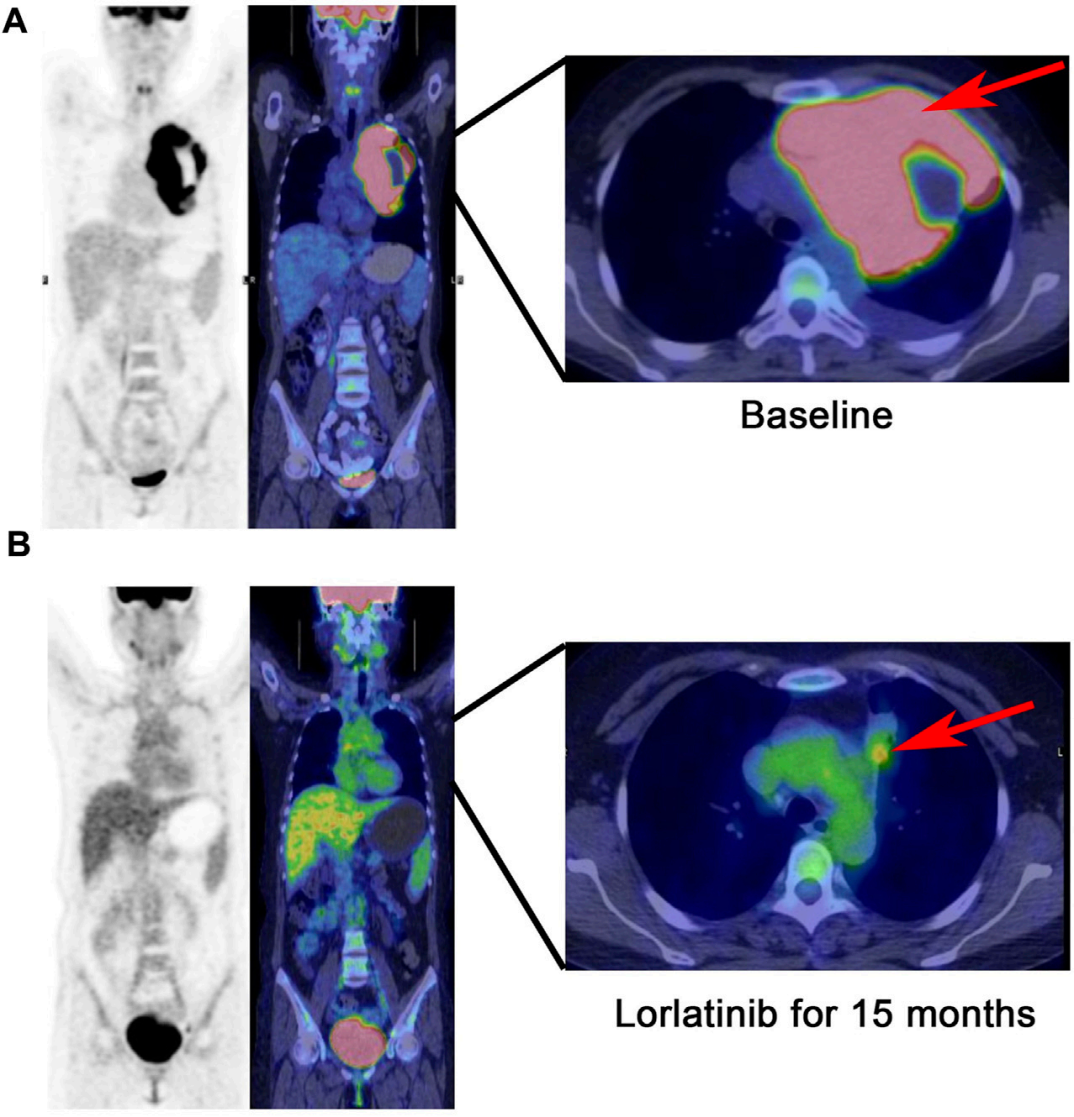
PET-CT scan: The tumor with a size of 14.8 * 9.9 cm is limited to the mediastinum without metastasis at baseline (A), continuous partial response was demonstrated after 15 months following lorlatinib (B).
